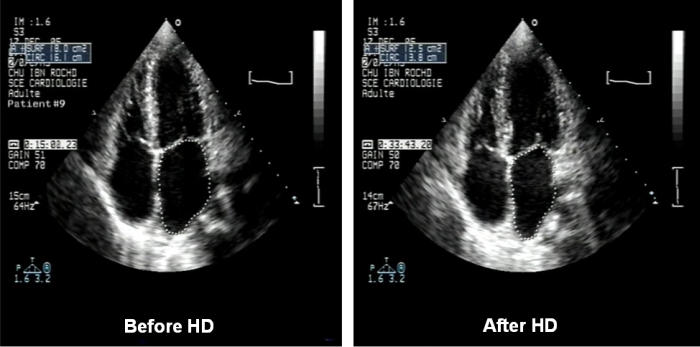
Echocardiogram (4-chamber view) of a patient before (left panel) and after (right panel) HD.
